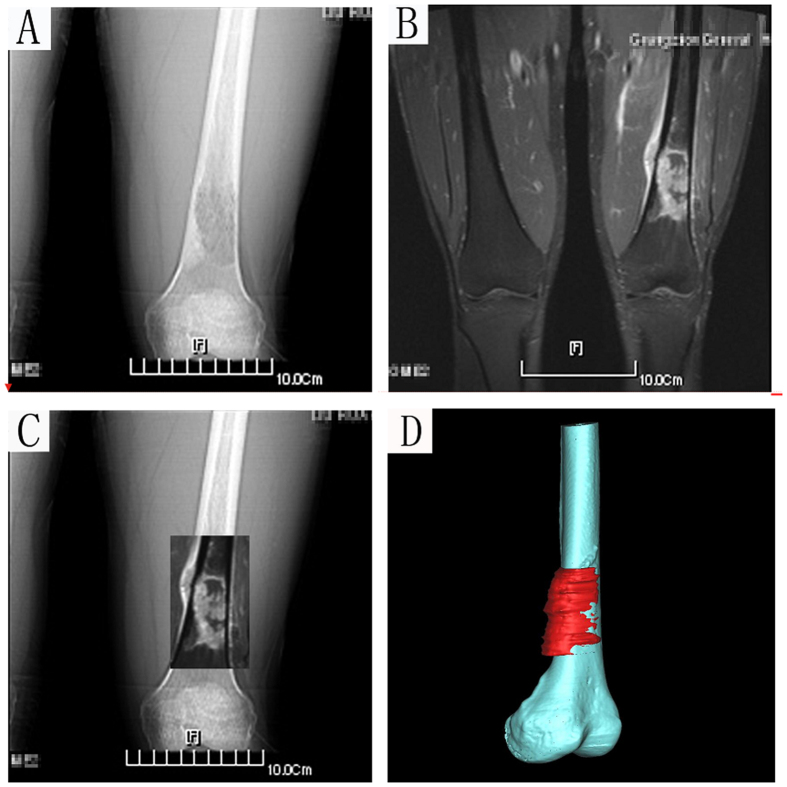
A 21-year-old patient with osteosarcoma of the distal femur. (A) Anteroposterior CT scan image. (B) MRI image. Both CT and MRI images revealed an osteosarcoma in the left distal femur. (C) Merging of the CT and MRI images for the delineation of the tumor. (D) The 3-D bone tumor model reconstructed from the merged CT and MRI image with the tumor profile highlighted in red.
